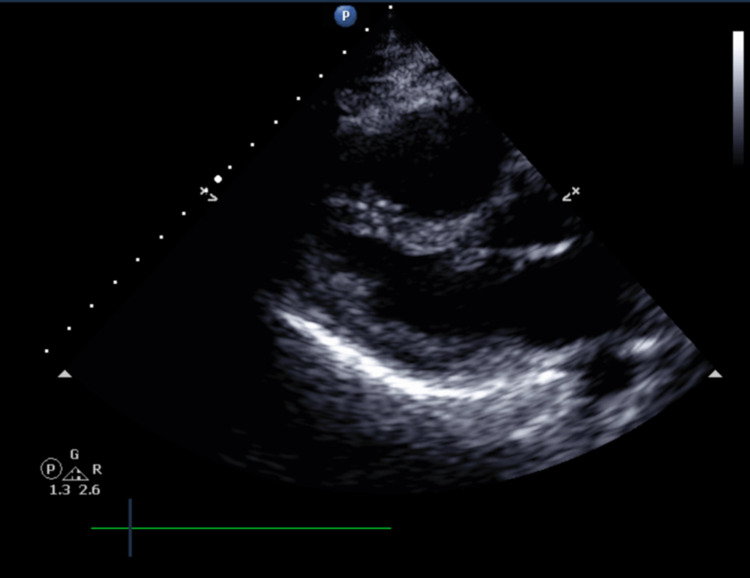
Transthoracic echocardiogram showed that right ventricle was dilated and severely hypokinetic with moderate tricuspid regurgitation
